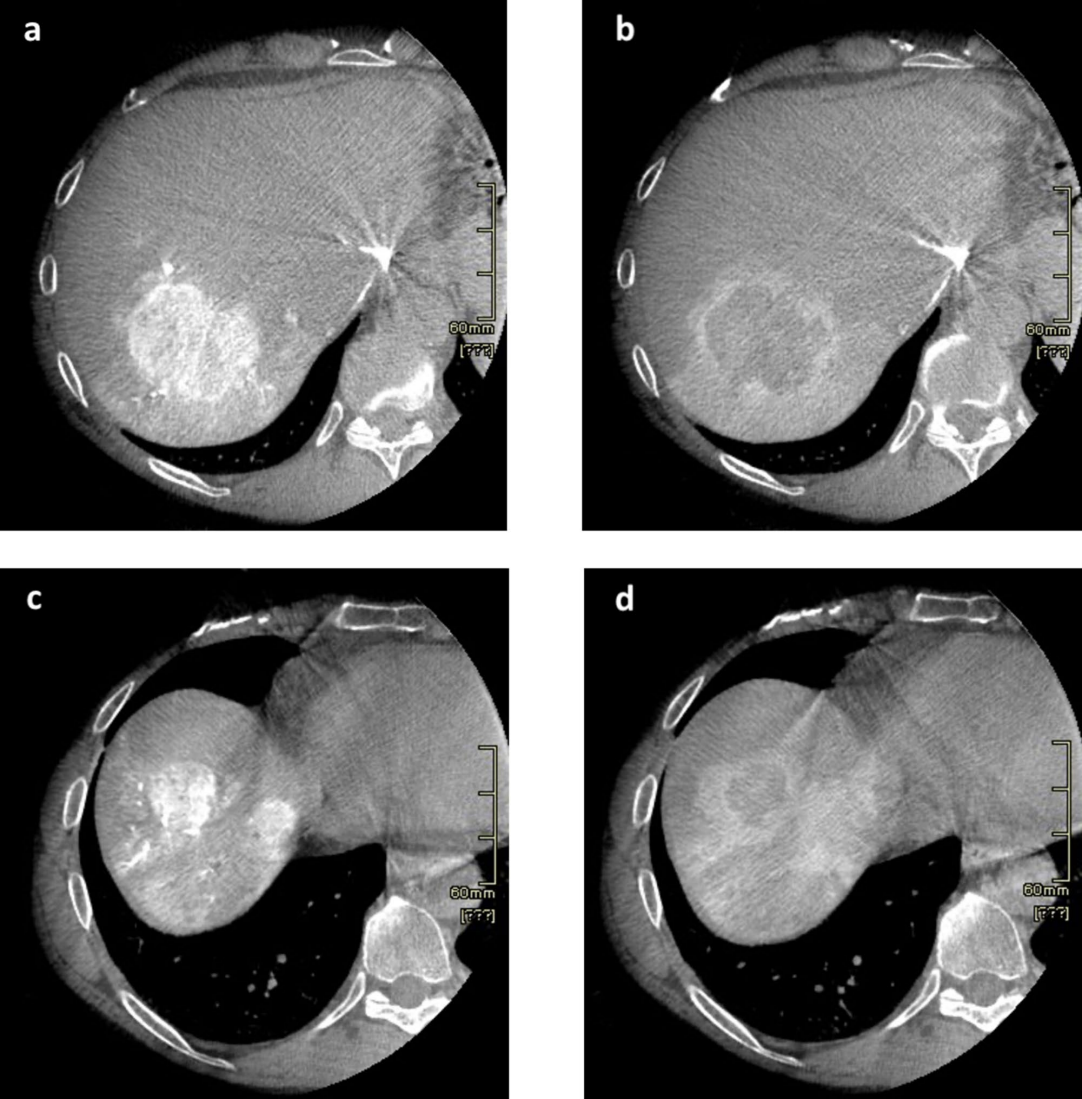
Dual-phase CBCT: a Early arterial phase (10–15 s) Lesion 1; b Late arterial phase (30 s) Lesion 1; c Early arterial phase (10–15 s) Lesion 2 and 3; d Late arterial phase (30 s) Lesion 2 and 3
